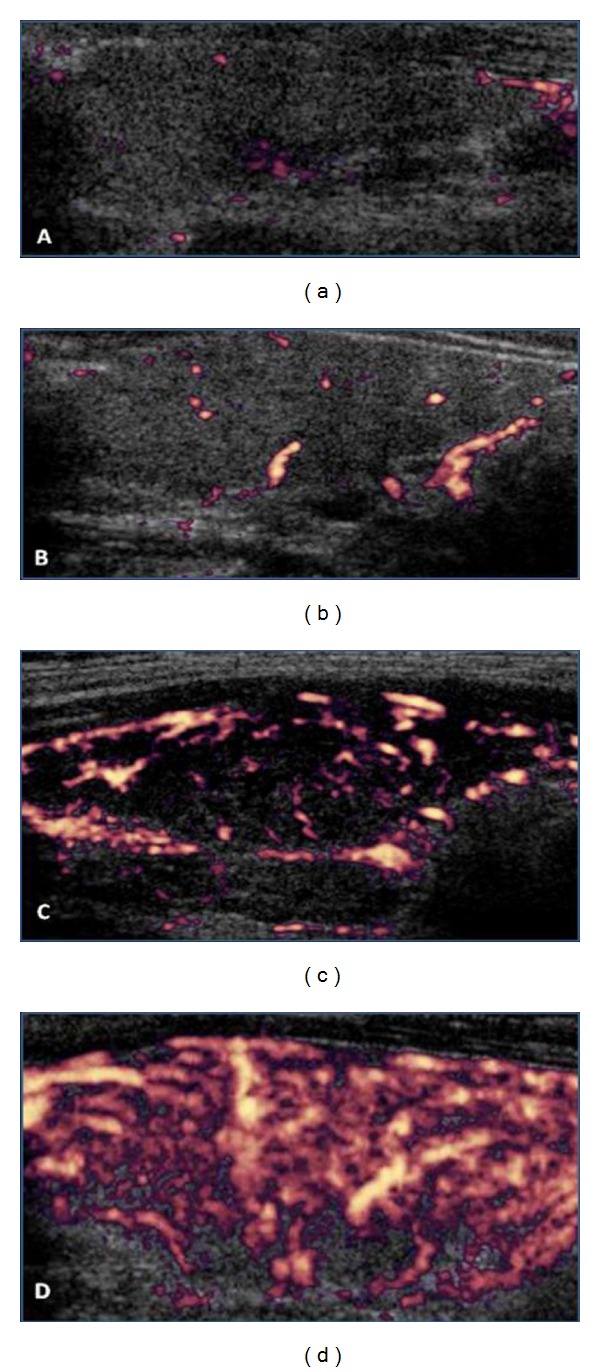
Power Doppler. Figures (a), (b), (c), and (d) correspond, respectively, to the vascularization patterns I, II, III, and IV of the thyroid parenchyma.
